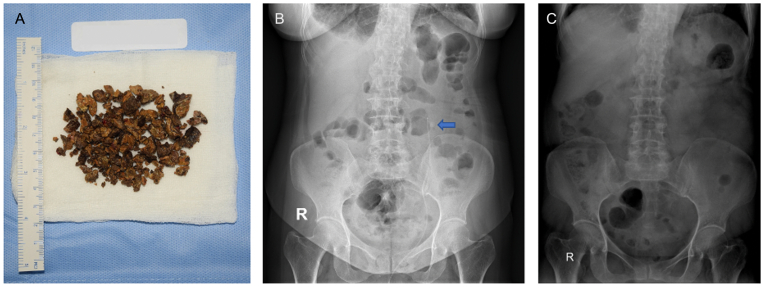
(A) Evacuated stone fragments, (B) postoperative kidney-ureter-Bladder X-Ray, (C) Post ESWL KUB X-ray.
